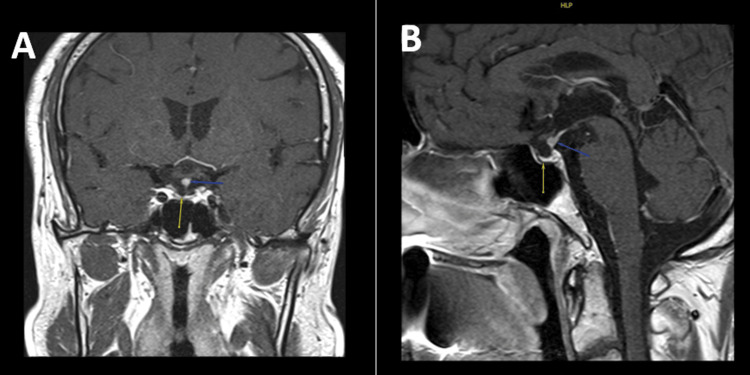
MRI pituitary protocol (A: coronal plane, B: sagittal plane) showed a focal, rounded area of widening seen involving the proximal part of the pituitary stalk, which showed homogeneous enhancement measuring around 4 mm.
